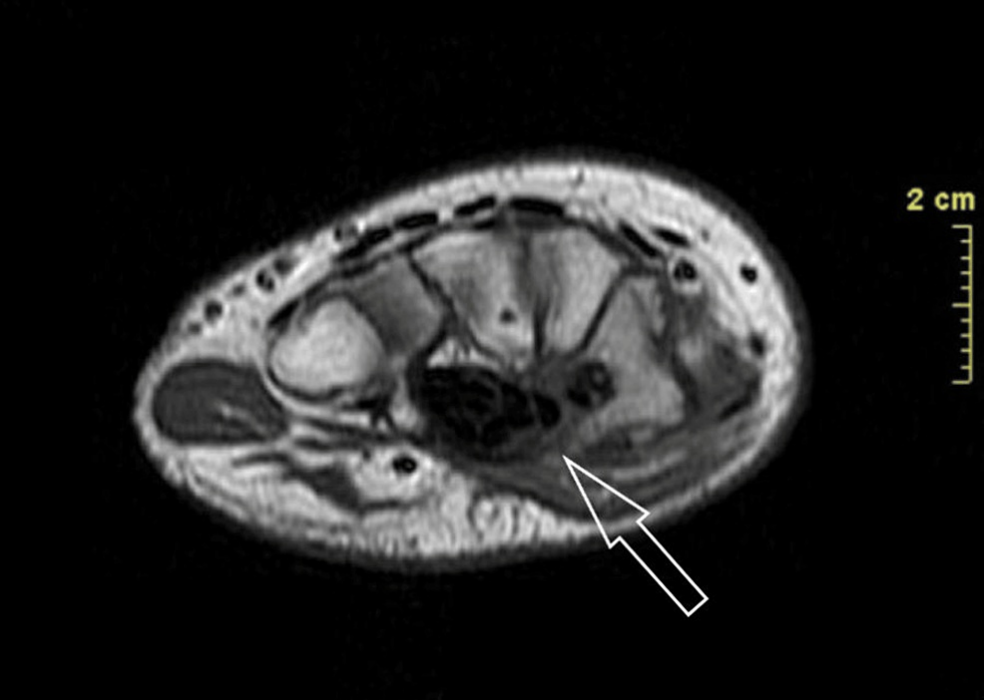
Wrist MRI MRI of the affected wrist. A hypointense lesion of the trapezium is visible.
